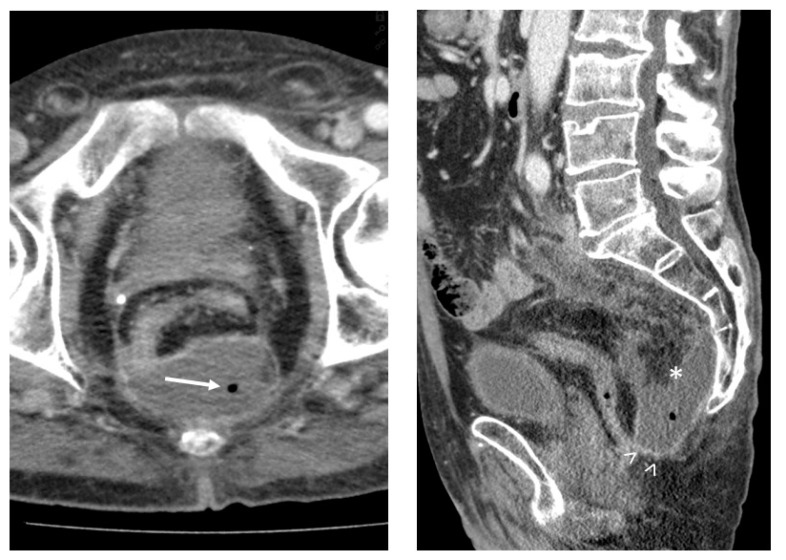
A 63-year-old man with presacral abscess (*), demonstrating gas entrapment (→), wall enhancement (>), fat stranding and CT attenuation of 29 HU. Microbiology after percutaneous drainage confirmed infection.
